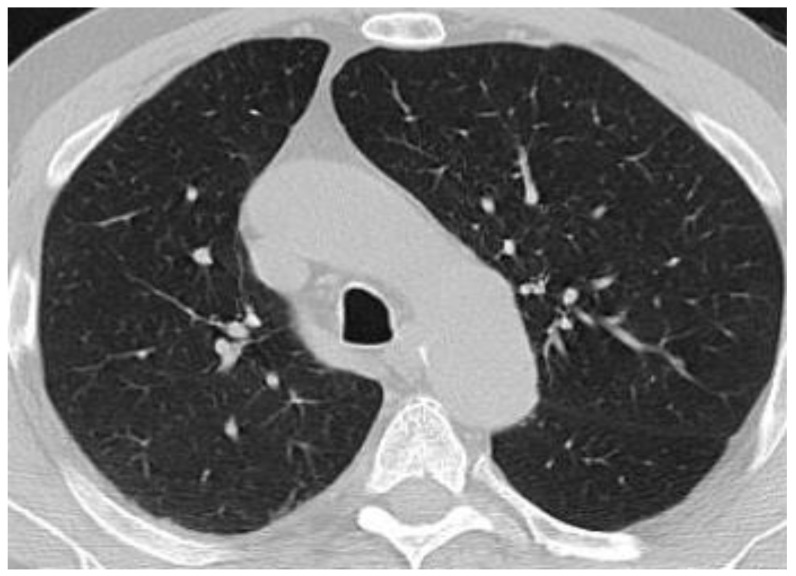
Computed tomography of the chest before immunotherapy.
